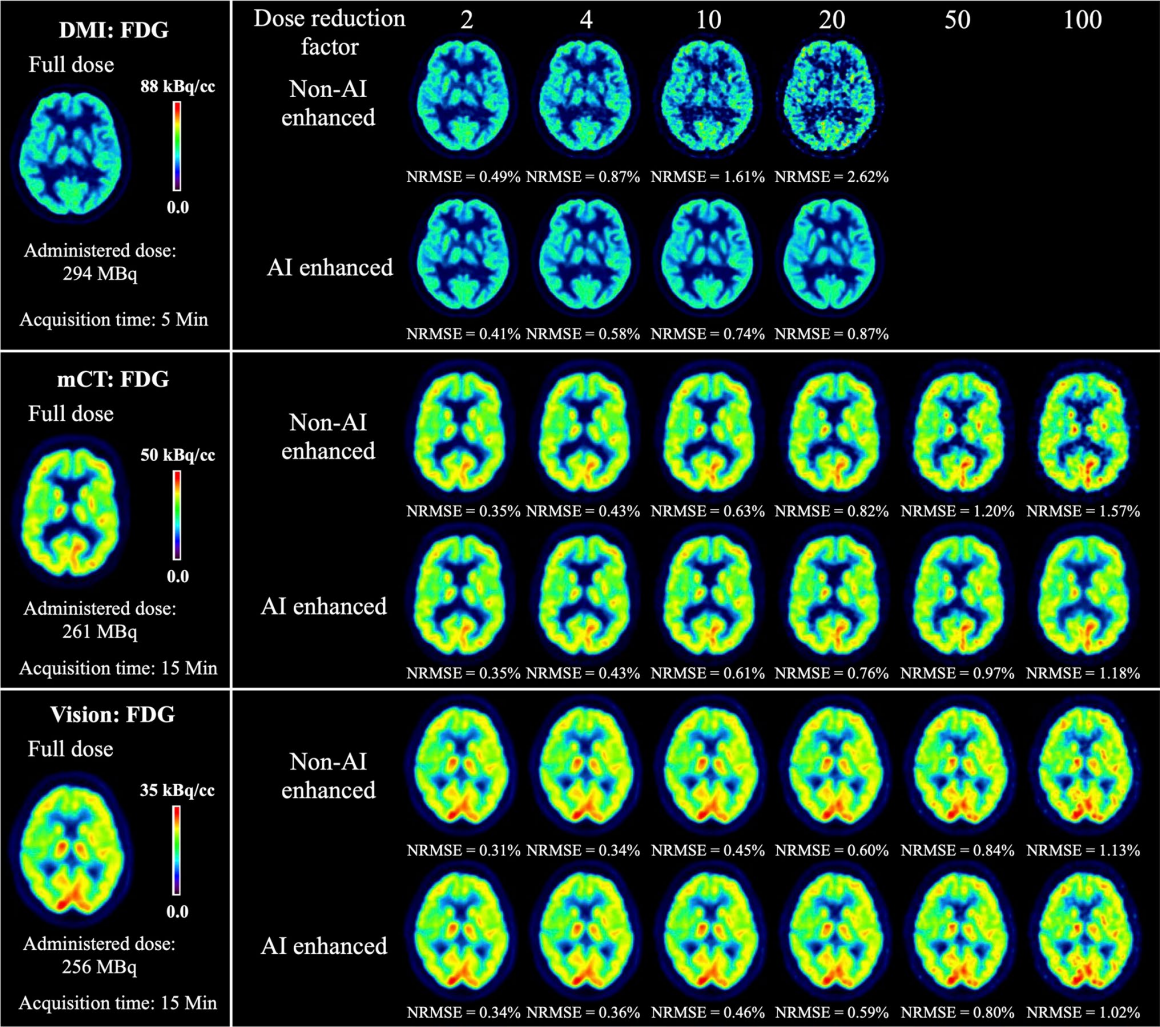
Example of test results of [^18^F]FDG imaging from DMI (GE Discovery MI), mCT (Siemens, Biograph mCT), and Vision (Siemens, Biograph Vision)
